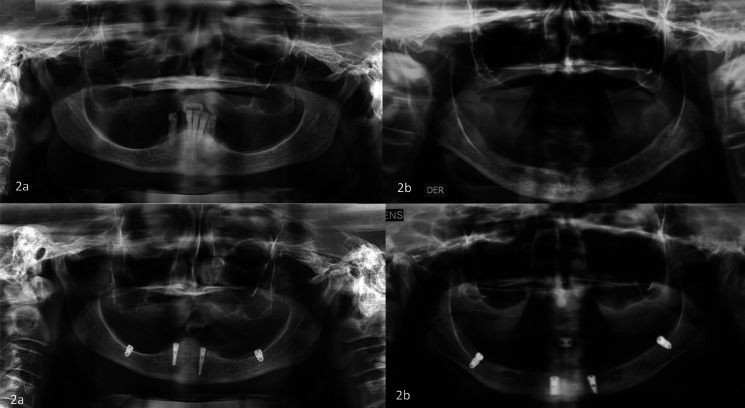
a) Radiographic image taken before and after implant placement. Clinical case. b) Radiographic image taken before and after implant placement. Clinical case.
